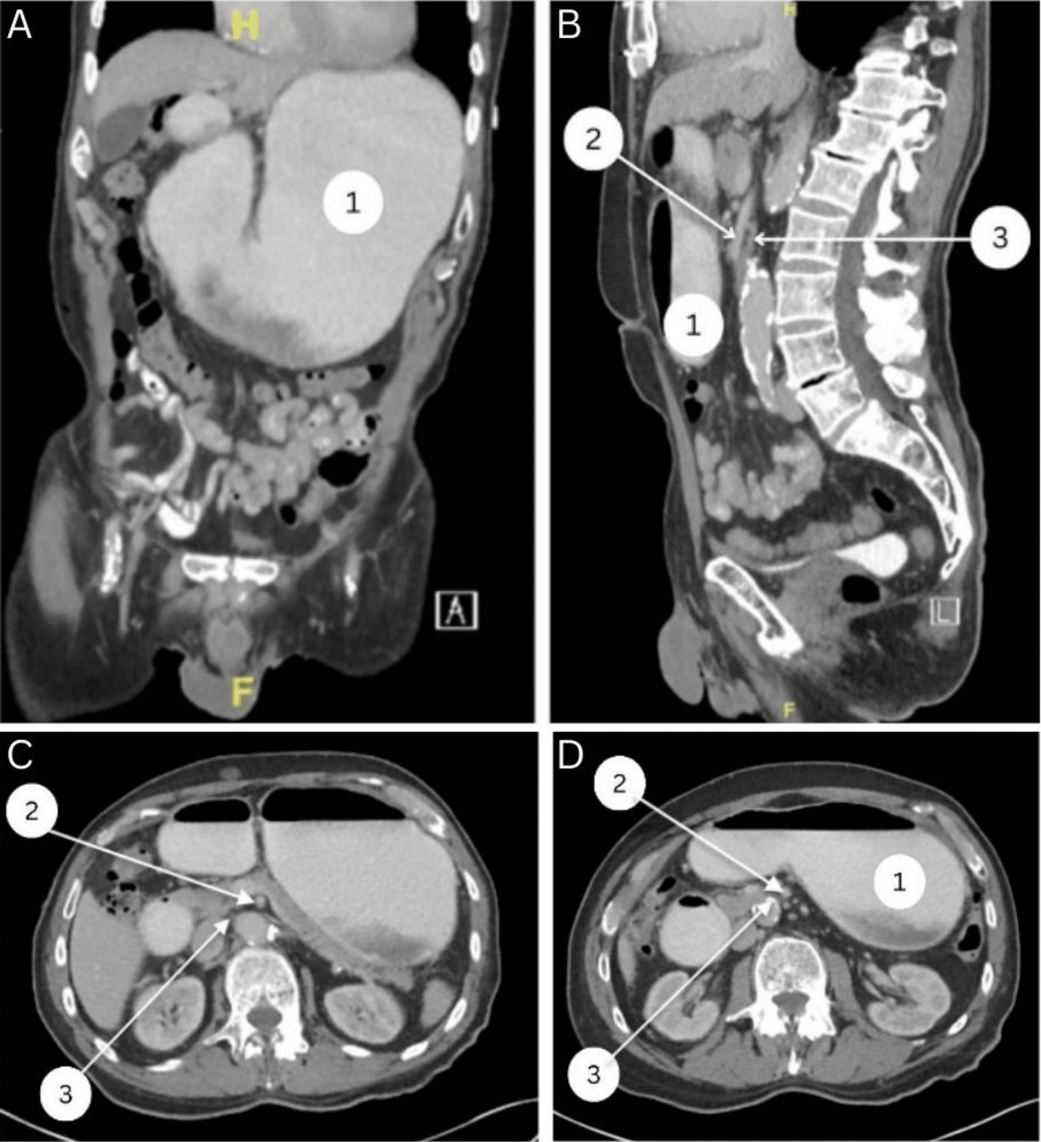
Preoperative CT images in the coronal, sagittal, and axial views (1) Distended stomach; (2) superior mesenteric artery; (3) compressed third portion of the duodenum
